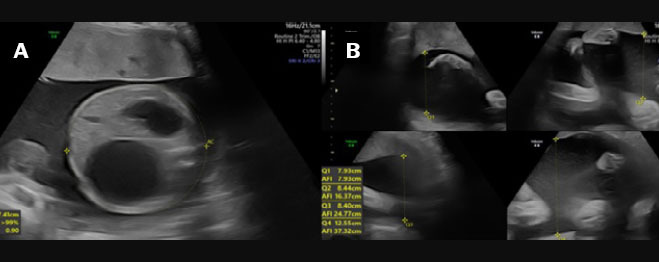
Two-dimensional ultrasound images, acquired with a convex 4–8 MHz
